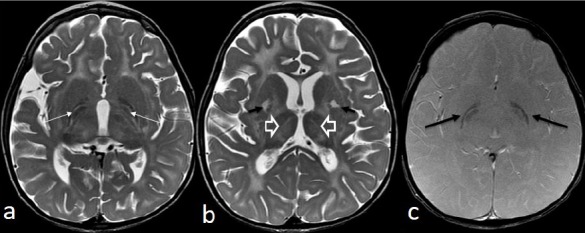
A brain MRI/MR axial (a and b) T2-weighted MR images show severe hypomyelination with a low signal of the globus pallidus within the medial and lateral segments separated by the hyperintense medial medullary laminae (white long arrows). The internal medullary laminae of the thalami are hyperintense (open arrows). A high signal intensity alteration is seen in both the putamina (short black arrows). The gradient echo weighted image (c) clearly shows the low signal of the globus pallidus (long black arrows)
